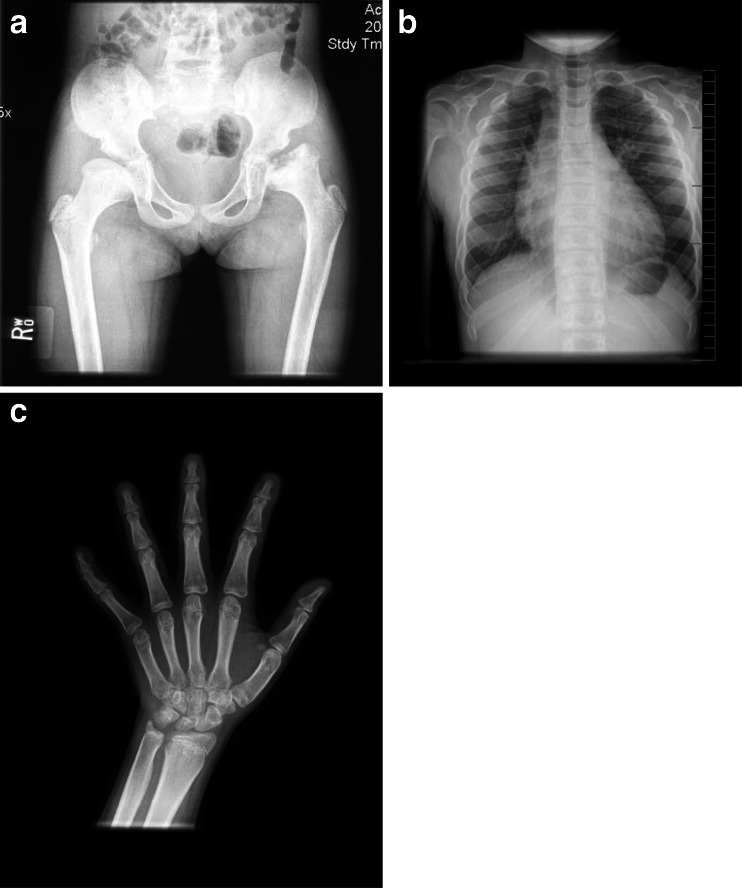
Radiographs of patient 7, female, MPS VI. a AP pelvis and hips, age 11 years: slightly abnormal pelvis with mild residua of inferior ileal tapering; bilateral CFE dysplasia with flattened epiphysis on the right and flat erosive changes on the left. b AP chest, age 11 years: the ribs are thick, but not paddle-shaped; clavicles are also thick. c AP hand and wrist, age 11 years: normal; no proximal pointing or brachydactyly; normal size/shape of carpal bones
